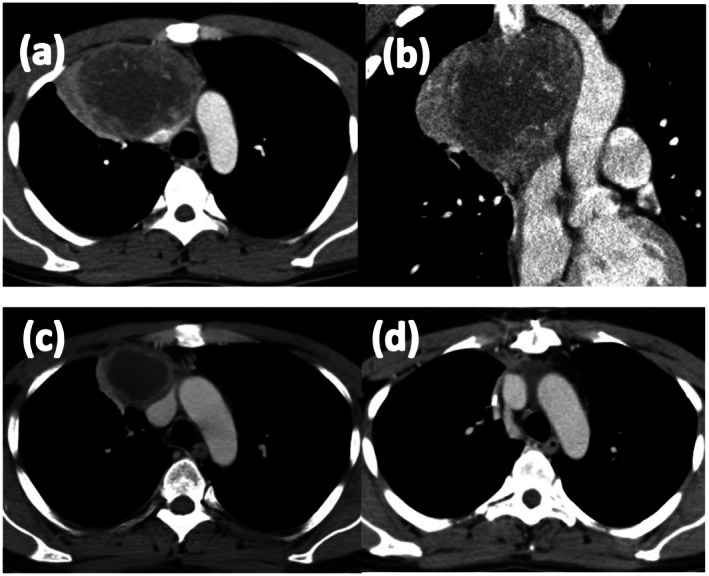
Contrast‐enhanced computed tomography (CT) images of the anterior mediastinal tumor. (a) Axial view at initial diagnosis reveals a 100‐mm solid mass in the anterior mediastinum. (b) Coronal view of the same lesion confirms its extent with no evidence of distant metastasis. (c) Axial view just before surgery shows a residual 55‐mm cystic mass. (d) Axial view after surgery demonstrates complete resection with no evidence of residual tumor.
